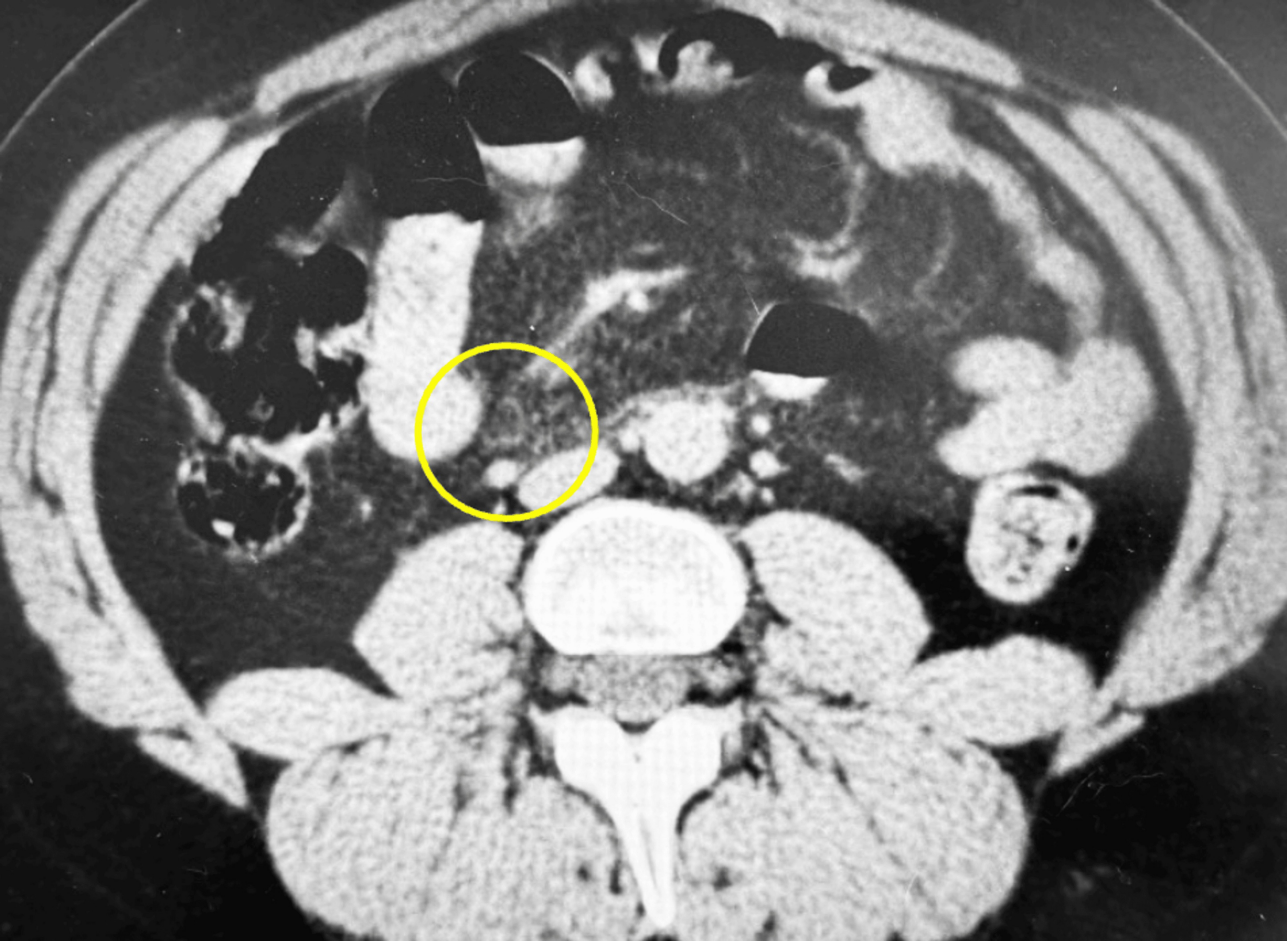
Axial CT image showing mesenteric panniculitis.
